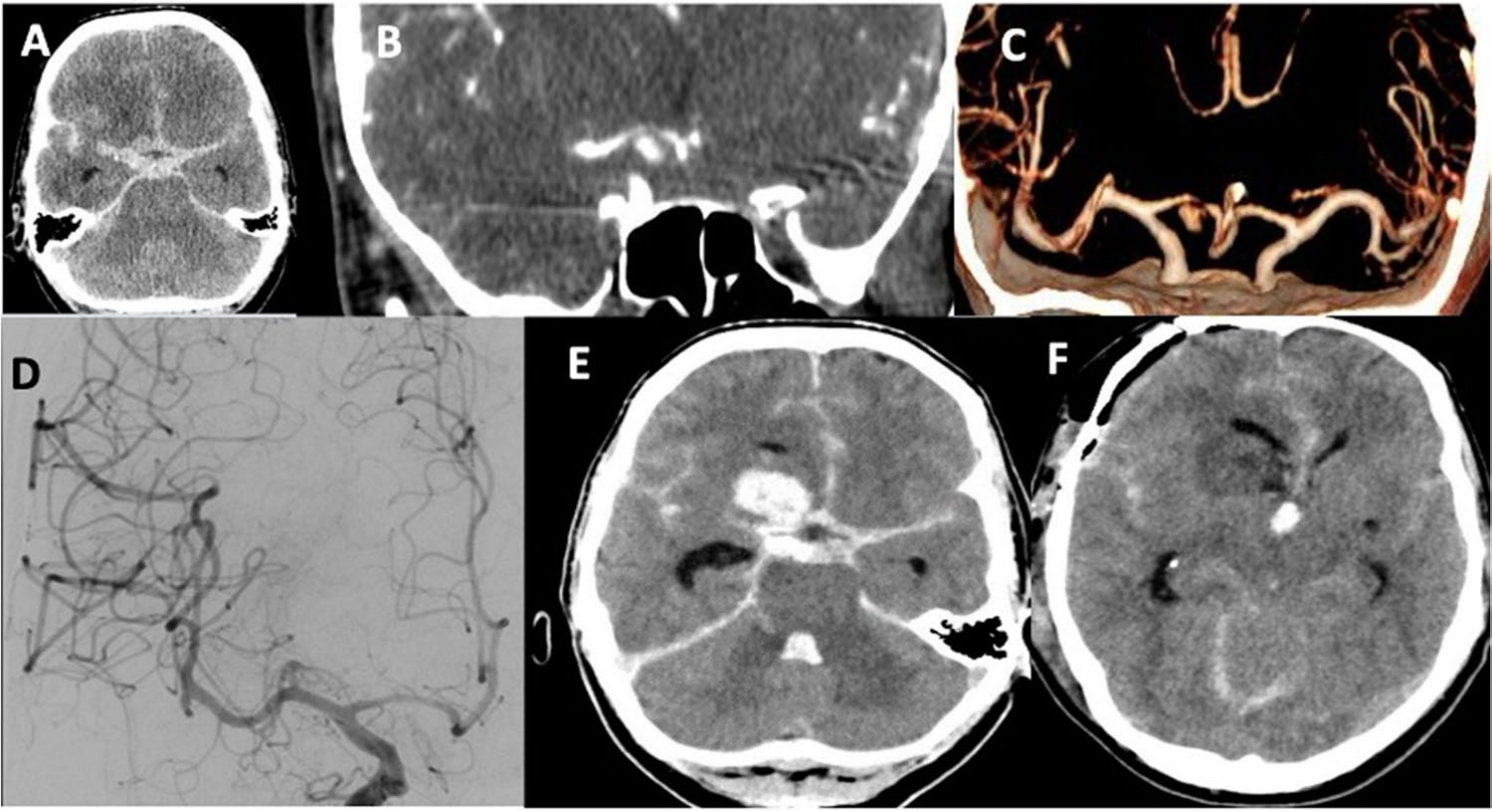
Case 1, 58-year-old woman. Computed tomography (CT) with axial reconstructions (A) performed immediately after the onset of severeheadache revealed a subarachnoid hemorrhage, Fisher grade 3, caused by the rupture of an aneurysm related to the right anterior cerebral artery (B, C). Transfemoral digital subtraction angiography (D), performed at the same day of symptoms onset, did not show the aneurysm. A control CT (E) immediately after the angiography revealed a new intracerebral bleeding in the right frontal lobe with intraventricular hematoma. The patient underwent emergency surgery, in which a large thrombosed fusiform aneurysm of Heubner’s artery with total occlusion of the parent artery was detected. Follow-up CT scans (F) showed an infarction in the right caudate nucleus near the frontal horn of the right lateral ventricle due to occlusion of Heubner’s arteryCase 2 (Fig. 3): A 67-year-old man with a history of arterial hypertension was admitted with severe headache, nausea and vomiting followed by right-sided hemiparesis und decreased consciousness. A cranial CT scan and CT-angiography (not shown) at admission revealed a SAH, Fisher grade 3, more prominent on the left than on the right side, with a prepontine blood clot in addition, without evidence of an aneurysm, accompanied by acute hydrocephalus. The patient underwent emergency placement of an EVD via a right frontal burr hole trepanation. On the same day, a transfemoral cerebral panangiography was performed which showed no evidence of a ruptured aneurysm. He was admitted to the neurointensive care unit, where he remained intubated and sedated. Five days after admission, he was successfully extubated. One week after the event, he presented with aphasia, prompting another cerebral angiography that revealed vasospasms in both middle cerebral artery territories. He underwent intraarterial spasmolysis using Nimodipine, which was repeated two more times. An MRI two weeks after the event revealed a small pontine infarction on the left side which was related to an occlusion of a perforator of the basilar artery. One month after the event, a control cranial CT scan clearly showed demarcation of the small left-sided pontine infarction. Five months after transfer to rehabilitation, the patient suffered from moderate disability, requiring some assistance but able he was able to walk unassisted.
